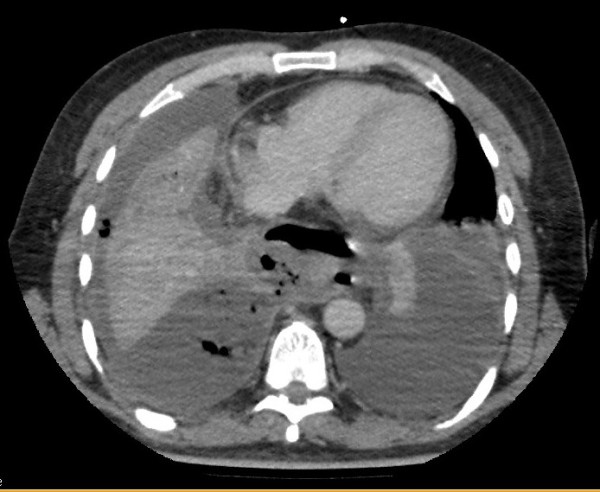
CT Thorax showing bilateral pleural effusions and contrast leak in right hemithorax.
